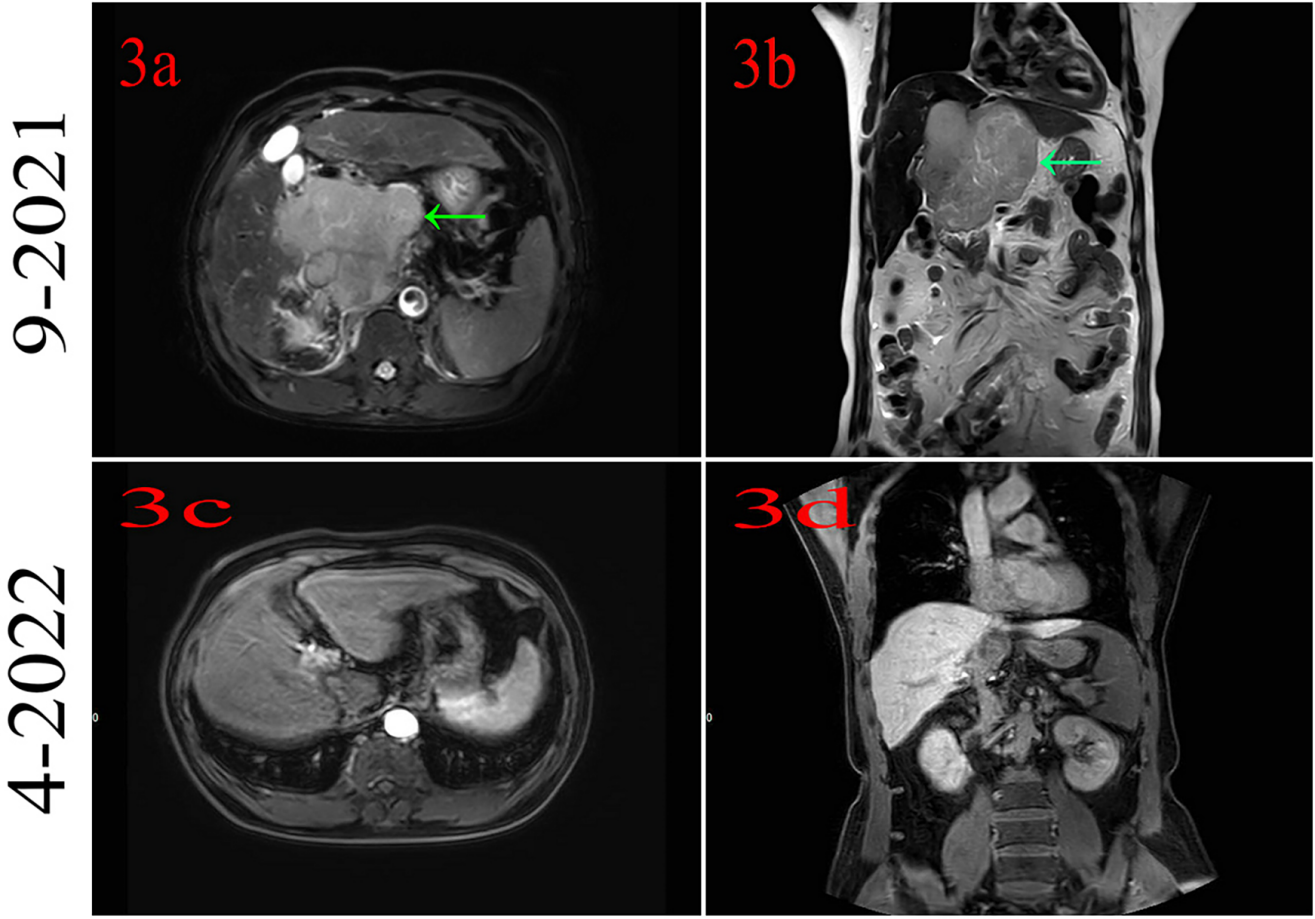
(a,b) Magnetic resonance imaging indicates hepatocellular cancer at segment 1. (c,d) MRI indicates that the patient had complete remission.
